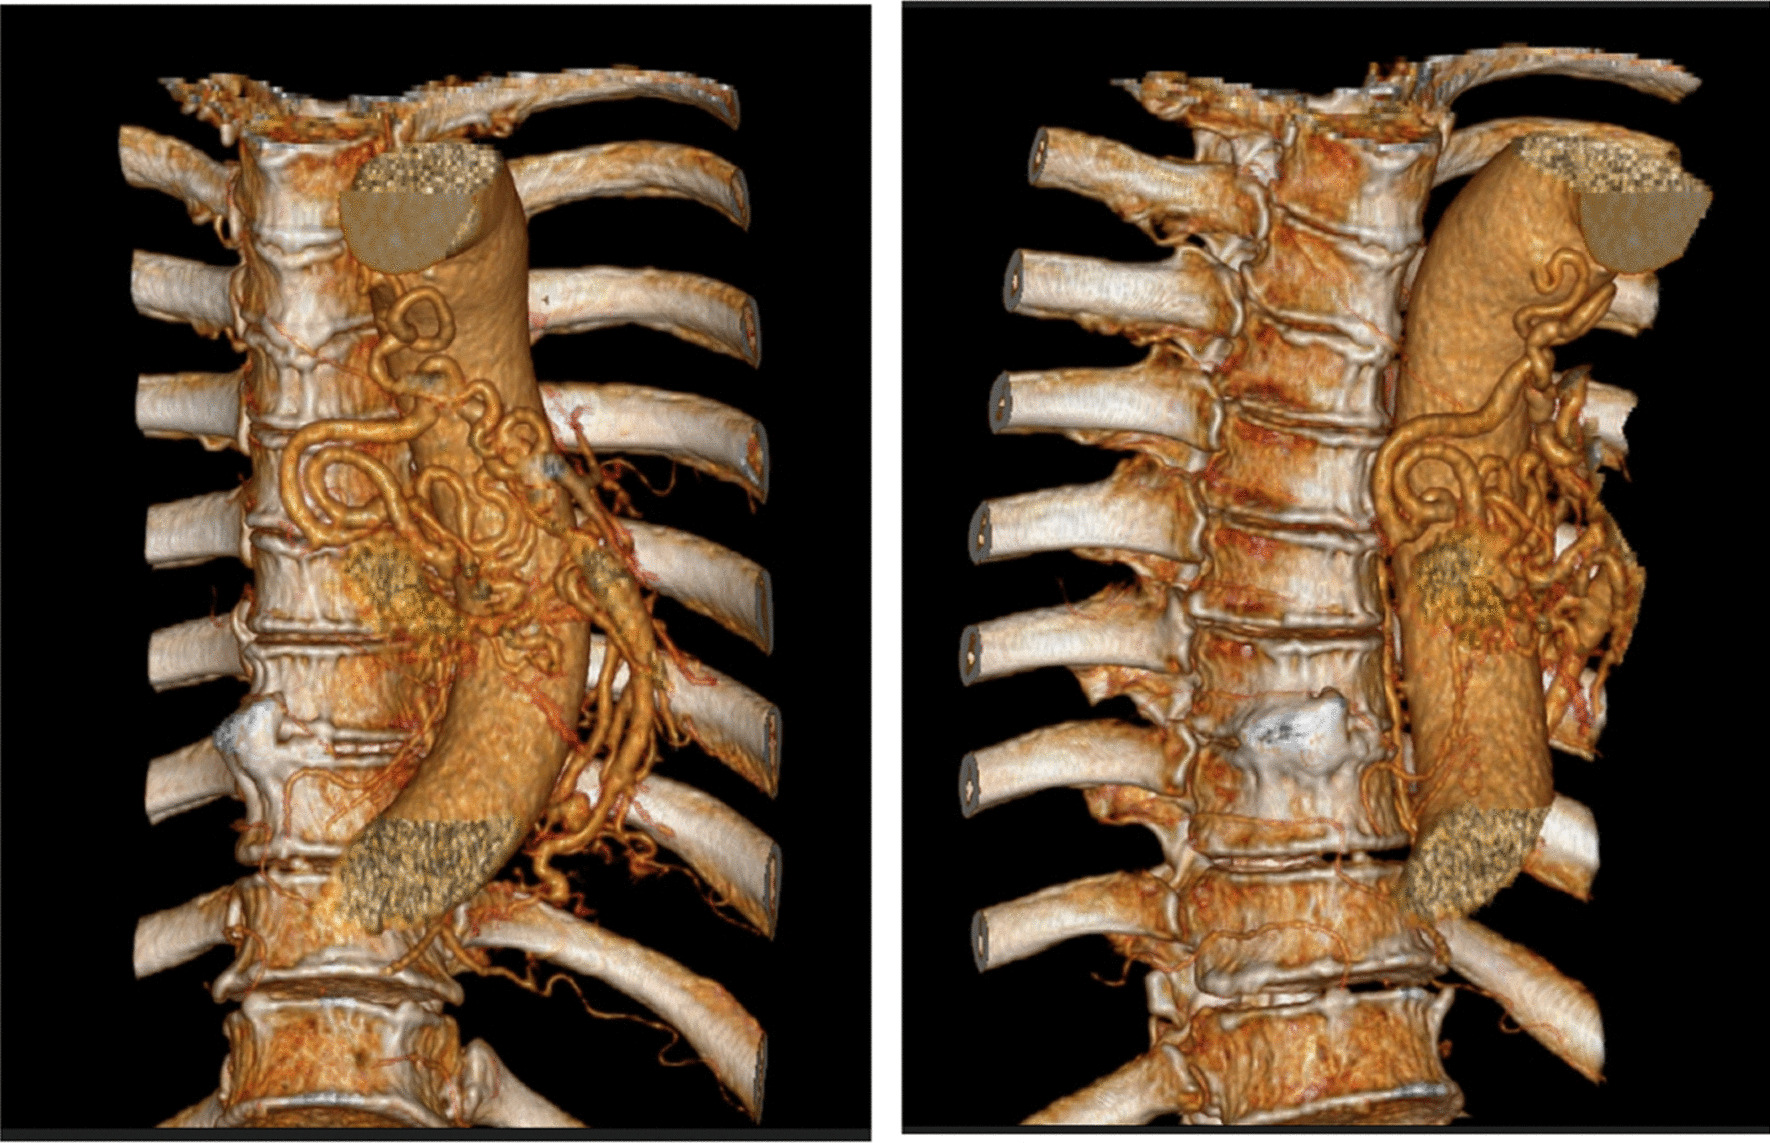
Computed tomography angiophy of bronchial artery (iterarive reconstruction): tortuous and dilated branches of left bronchial artery
